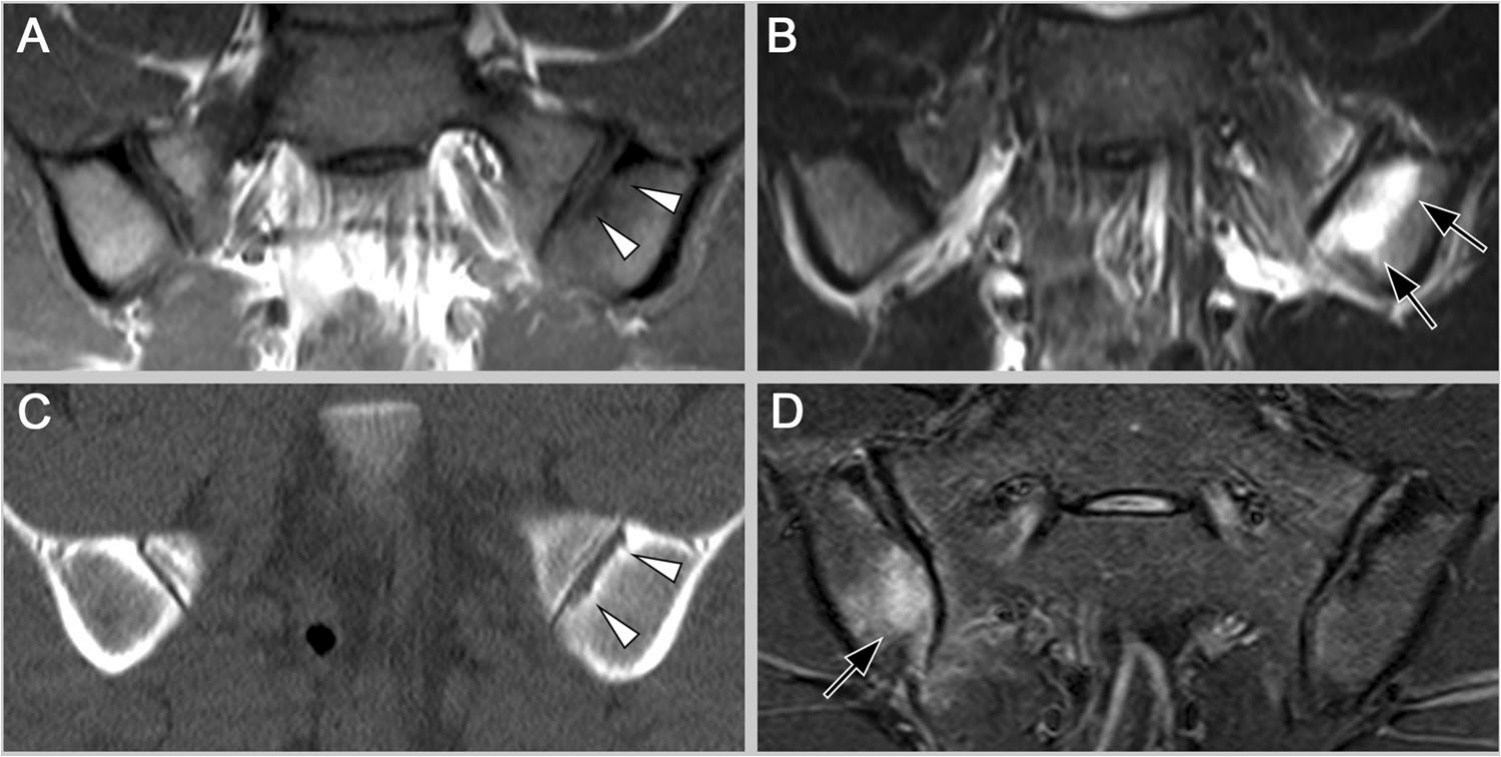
Mechanical stress or axSpA? This 28-year-old male athlete presented with inflammatory back pain. In the initial MRI, T1 (A) showed no unequivocal erosion (arrowheads). However, STIR (B) showed active inflammation in a region typical for mechanical stress reactions (arrows). With this uncertainty, the patient underwent ldCT (C) that confirmed erosion of the articular surface (arrowheads), and thus, the diagnosis of axSpA could be established. Follow-up MRI (D) after 2 years with new bone marrow oedema (arrow) further increased the confidence in this diagnosis
